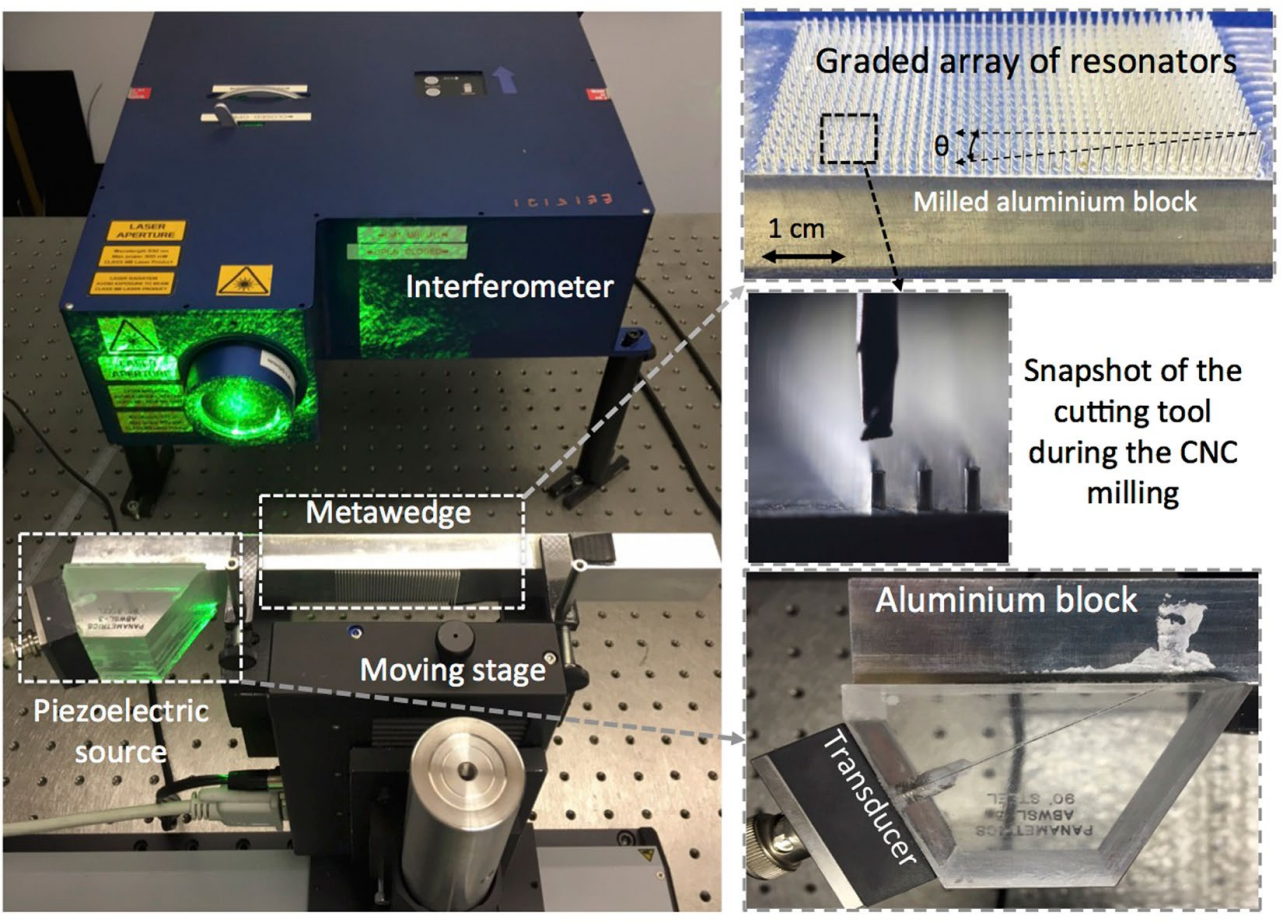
The experimental set-up consisting of a laser interferometer scanning the surface of the metawedge and the aluminium block mounted on a moving stage. The excitation is obtained with an ultrasonic transducer coupled with a 65° wedge that, by phase matching, filters out all incident waves except Rayleigh waves. The resonators have a 0.5 × 0.5-mm cross-section and an increasing (or decreasing) height from 0.5-mm to 3-mm and have been obtained by micro-milling of the surface.
